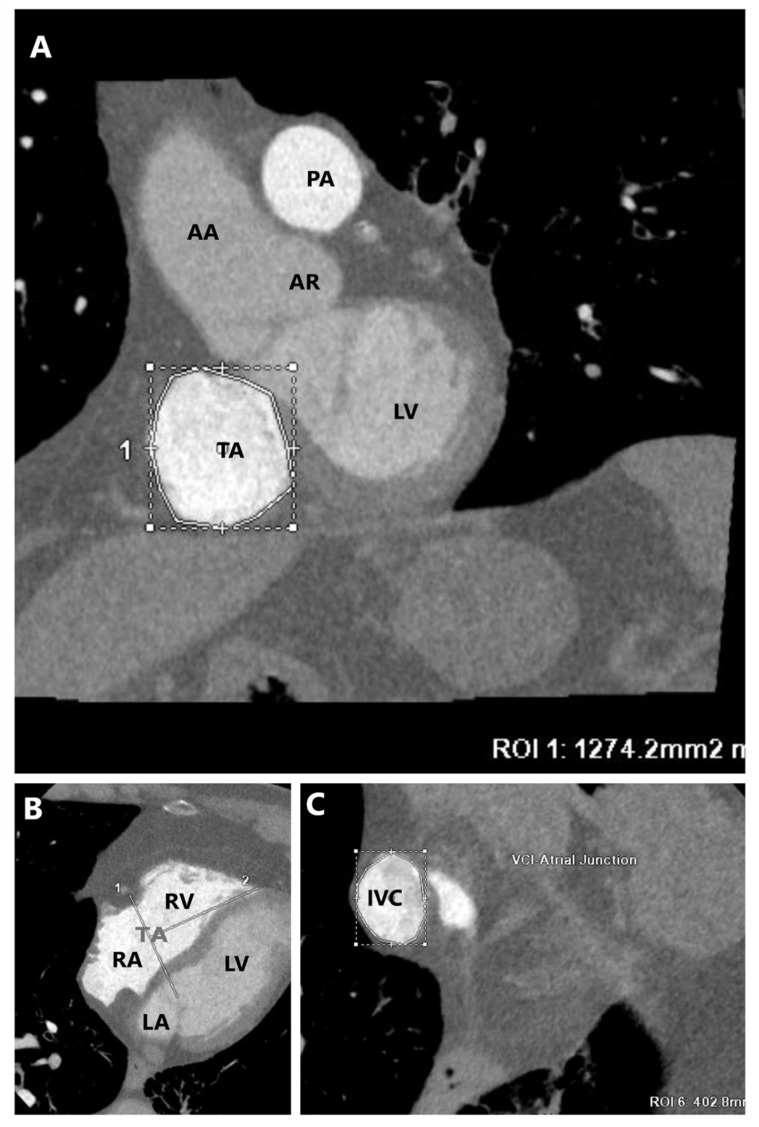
Assessment of TA dimensions by Cardiac Computed Tomography performed with a specific right–sided opacification protocol and by using multiplanar planes. (A) A planar cross-sectional area (short-axis) of TA that allows measurements of the TA area, perimeter, anteroposterior, and septal- lateral diameters. (B) Right-chambers morphology for the measurement of distance between the TA and the RV apex. (C) A transverse plane of the IVC. TA = tricuspid annulus, LV = left ventricle, AR = aortic root, AA = ascending aorta, PA = pulmonary artery, RA = right atrium, RV = right ventricle, LA = left atrium, IVC = inferior vena cava.
